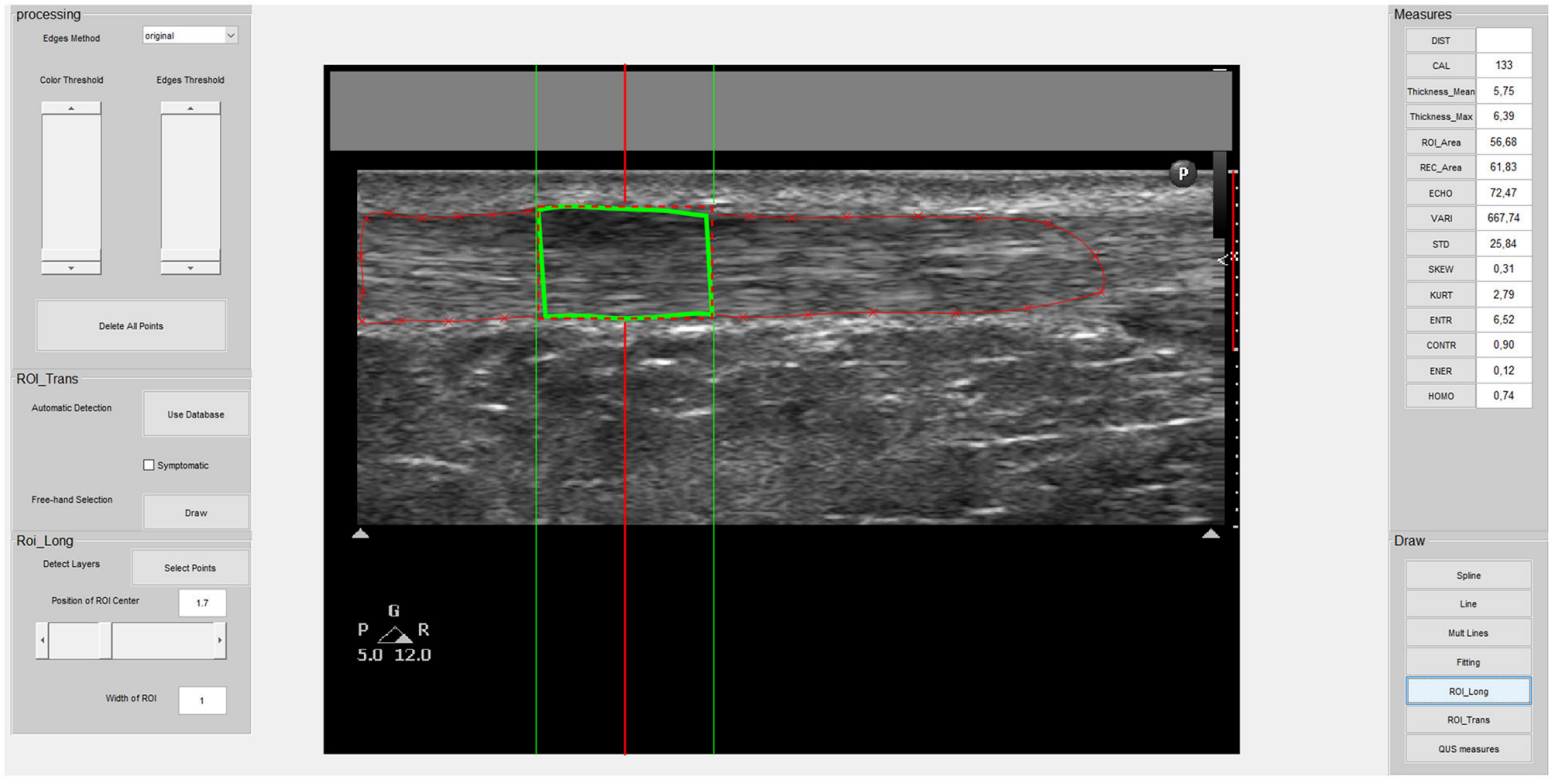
Screenshot of the custom-made program interface used to select the ROI and acquire musculoskeletal ultrasound biomarker (MUB) measures of the Achilles tendon image. This is an example of a symptomatic tendon in the longitudinal plane with a 1-cm wide ROI.
